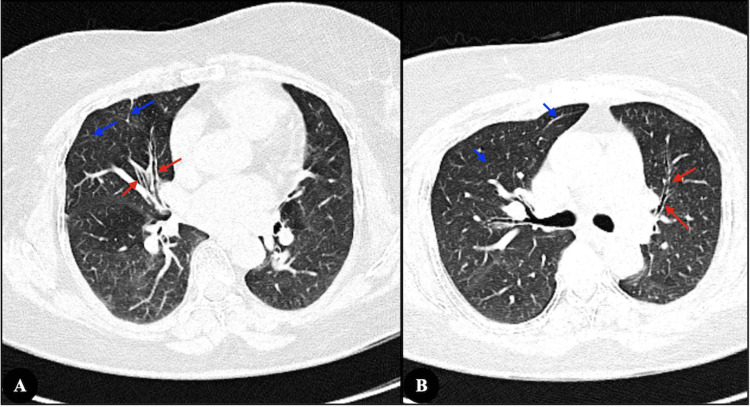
High-resolution computed tomography of the chest (A) Traction bronchiectasis in the medial segment of the right middle lobe (red arrows) with adjacent fibrotic streaks (blue arrows); (B) Traction bronchiectasis in the superior lingular segment of the left upper lobe (red arrows) with associated fibrotic bands (blue arrows)
